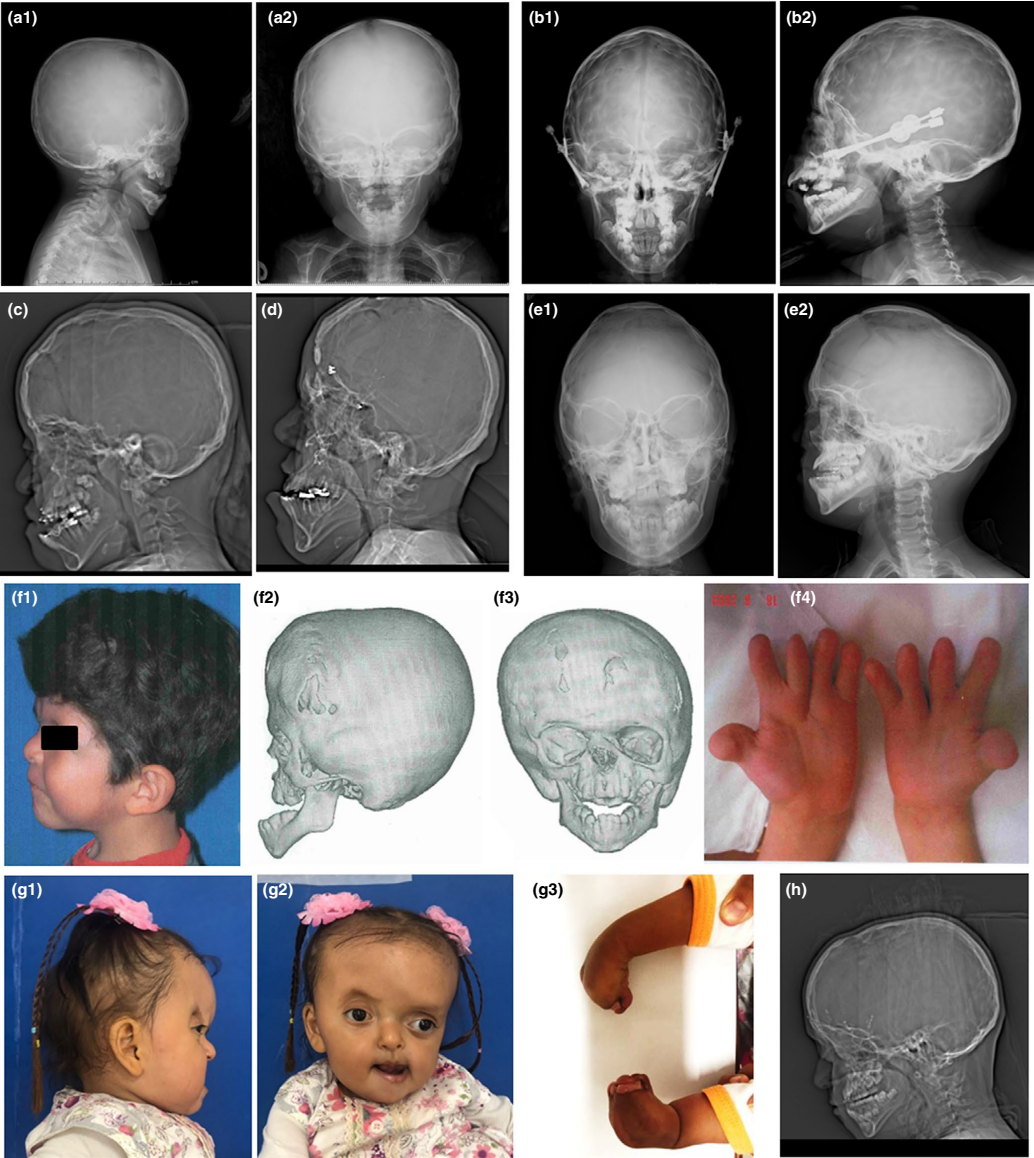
(a) Two‐year‐old male patient with Apert syndrome (AS‐5), skull radiography study in lateral (a.1) and anteroposterior (a.2) projection, showing: coronal synostosis, widening of the sagittal suture, retrusion of the midface hypoplasia, shallow orbits, digital printing at the temporoparietal level, and decrease in anteroposterior diameter. (b) Seven‐year‐old male patient with Crouzon syndrome, postoperative, with anteroposterior (b.1) and lateral (b.2) skull radiography. Findings: remodeling with widespread digital impressions suggestive of intracranial hypertension, distracting of the middle facial third. (c) Ten‐year‐old female patient with Crouzon syndrome, image of the lateral topography where it is observed: decrease in the anteroposterior diameter with a discreet increase in the craniocaudal diameter, digital impression, and retrusion of the midface hypoplasia. (d) Twenty‐six‐year‐old male patient with Apert syndrome (AS‐1), lateral topogram image showing: anteroposterior decline, postorbital frontal advance change, and severe retrusion of the midface hypoplasia. (e) Eight‐year‐old male patient with Crouzon syndrome, with anteroposterior (e.1) and lateral (e.2) cranial radiography, showing: postorbital frontal advance changes, retrusion of the midface hypoplasia, with irregularities in the cranial vault. (f) Eight‐year‐old male patient with Apert syndrome (AS‐2), flat facial profile, well‐shaped atrial canopy with low implantation (f.1), with 3D reconstruction images of CT scan of the lateral (f.2) and anterior (f.3) skull, where a decrease in the anterior posterior diameter of the skull is observed, wide forehead. In the frontal image, asymmetric orbits are observed, with smaller size of the left orbit and displaced below it, associated with midface hypoplasia, plagiocephaly, and prognathism. In the hand (f.4), short fingers (brachidactyly) with surgical scar are observed by correction of syndactyly. It presents cutaneous syndactyly and first toe with lateral displacement. (g) Three‐year‐old female patient with Apert syndrome; lateral (g.1) and frontal (g.2) photography, coronal closure of the left side is observed; type III syndactyly is present in the hands (g.3). (h) Eight‐year‐old male patient with Crouzon syndrome; image of lateral skull topogram where changes are observed postadvance fronto‐orbital with harmonic anteroposterior diameter and retrusion of the middle facial third
